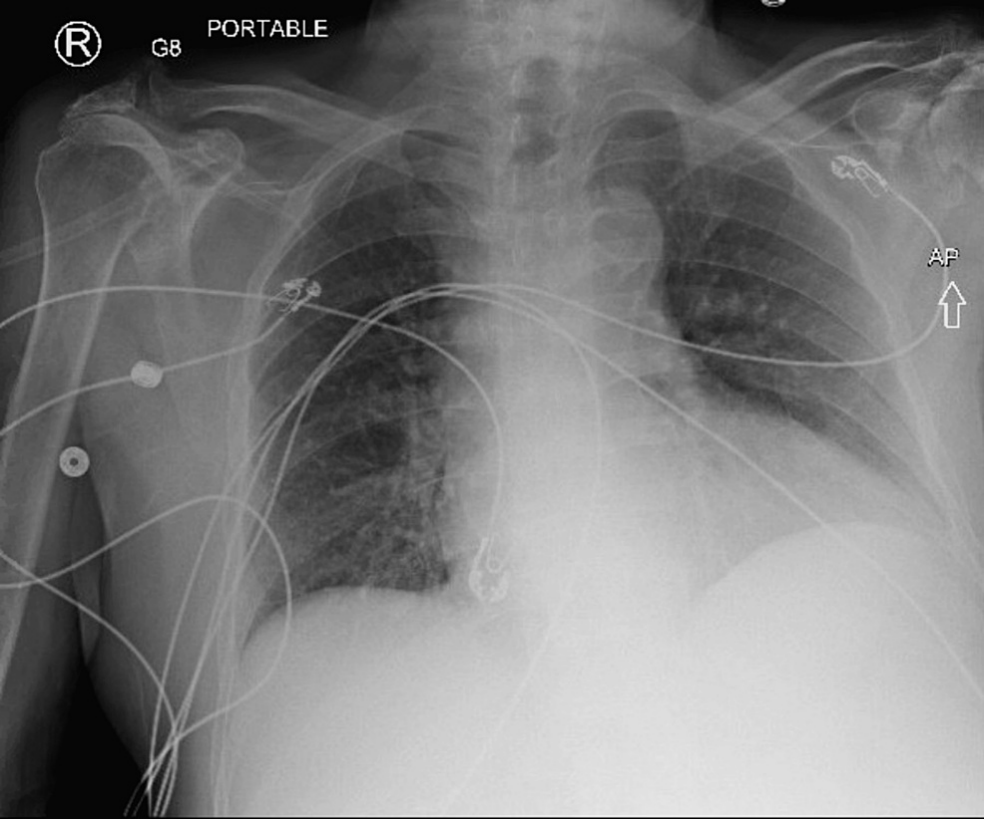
AP portable chest X-ray showing enlarged heart and low lung volumes AP: Anteroposterior
